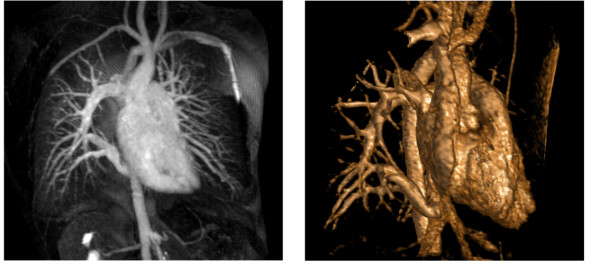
Pre- and postoperative MRI. 4.a) Preoperative MRI showed dextroposition and mesocardia, lower right pulmonary vein connecting to the inferior vena cava. Right upper and middle pulmonary veins draining in the superior vena cava and the azygos vein. 4.b) Postoperative MRI revealed the superior vena cava draining into the right atrium. The upper and middle pulmonary veins as well as the scimitar vein are redirected with a baffle into the left atrium.
